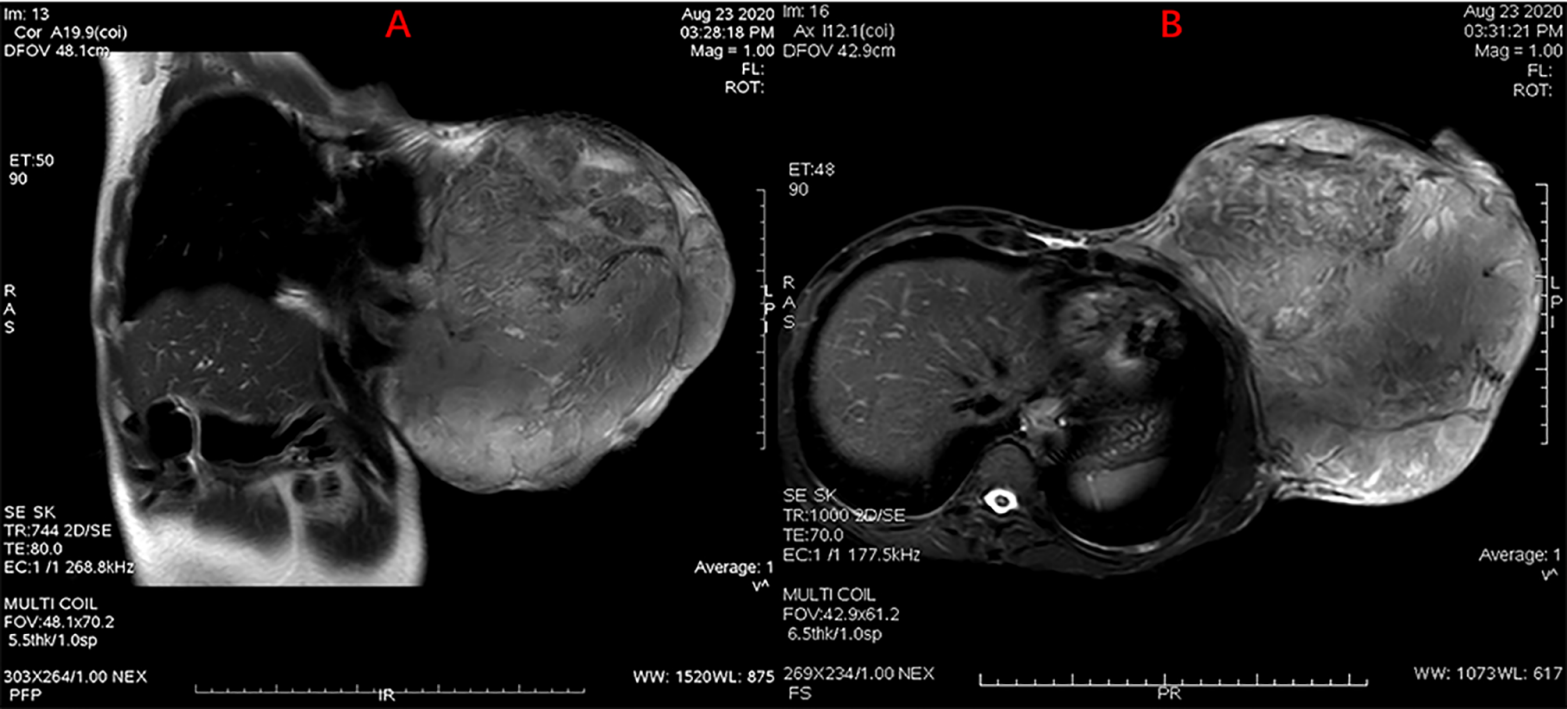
MRI scan showing a giant mass in the left breast with skin ulceration. Without chest wall infiltration or axillary lymph node metastasis were observed. (A) Sagittal MR scanning, (B) Horizontal MR scanning.
